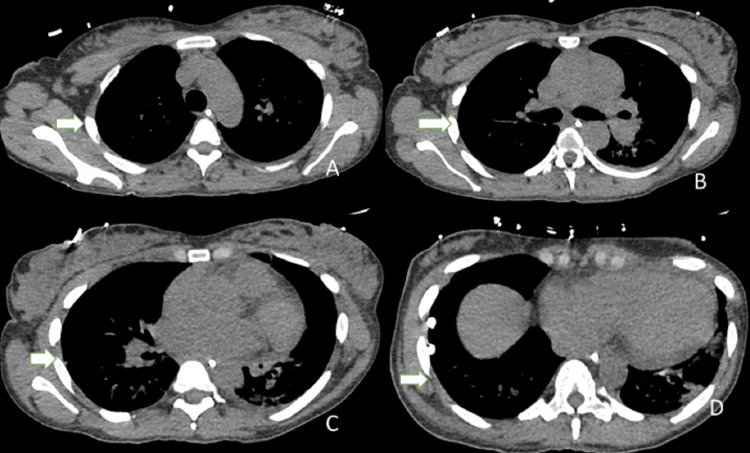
A follow-up CT of the chest after eight days of chest tube placement Mediastinal windows and resolution of right pleural effusion (white arrows). A. upper lobes; B. carina; C. middle lobe; D. lower lobes
